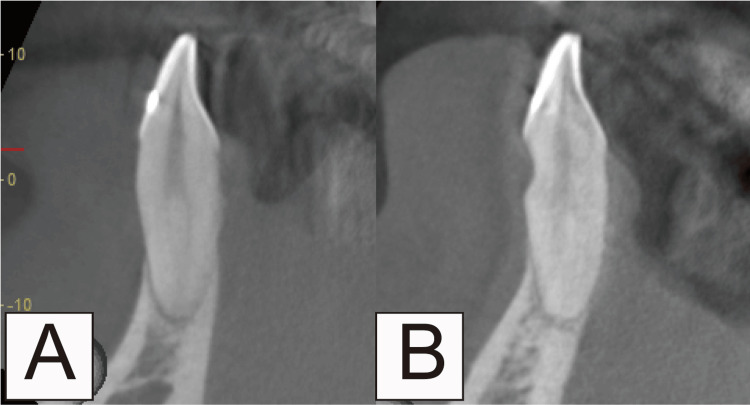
Flattening in a case of cervical dentin defect shown by cone beam computed tomography Cutting is performed to ensure the missing tooth structure and root flattening area are smooth. If there is no tooth loss in the cervical region, the tooth is designed and cut to be smooth in the area where root flattening is performed. In cases wherein the root shape is deformed, or the tooth is tilted and flattening is difficult to determine, a cone beam computed tomography scan should be performed. (A) Before root and cervical margin flattening procedure; (B) After root and cervical margin flattening procedure.
